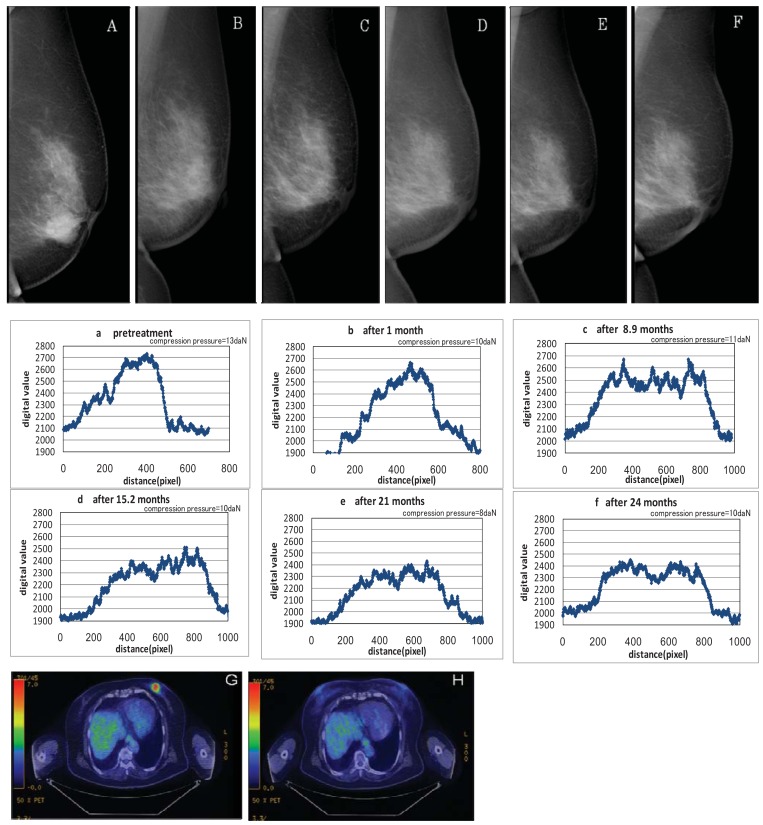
Case 2. Aged patient (79F) with left breast cancer (cT2N0M0) treated with radiosensitization (KORTUC II). A ∼ F: Pre and post-KORTUC II treatment evaluation of changes of tumor shadows on MMG. a ∼ f: Profile curve of changes of tumor shadows (1 pixel = 93.9 μm). G, H: PET-CT images before KORTUC II (G) and 13 months after KORTUC II (H). (G) Tumor diameter is 22 mm (SUV_MAX_ = 7.4). (H) There is neither local recurrence nor distant metastasis.
